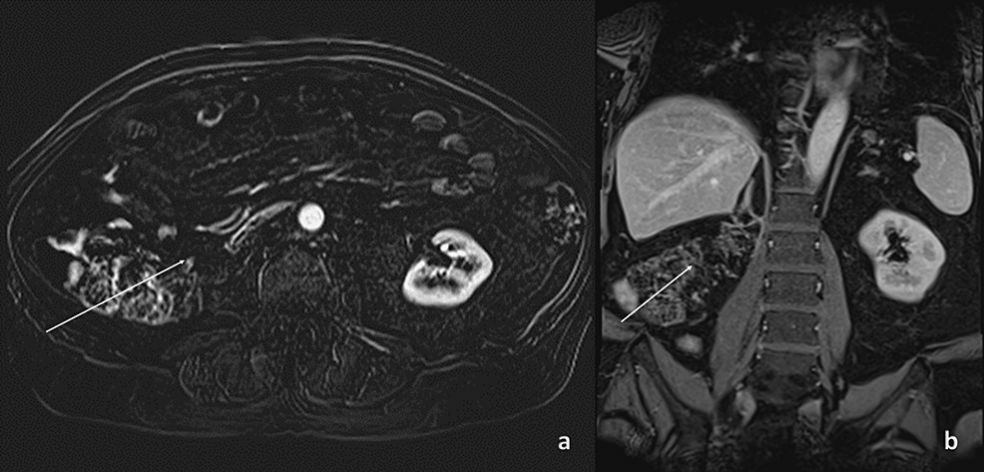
Follow-up MRI of the abdomen at three months after the initiation of therapy Post-radical right nephrectomy showed no convincing residual or recurrent tumor evident on axial (a, arrow) and coronal (b, arrow) sections. MRI: magnetic resonance imaging
